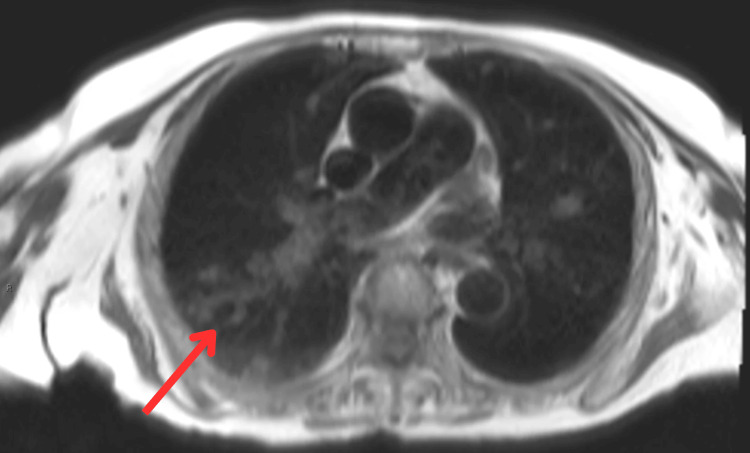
Cardiac MRI: axial T1 image at the level of the main pulmonary artery. The image demonstrates T1 intermediate-signal intense intraluminal lesions in the right pulmonary artery and posterior segmental pulmonary artery of the right upper lobe (red arrow).
